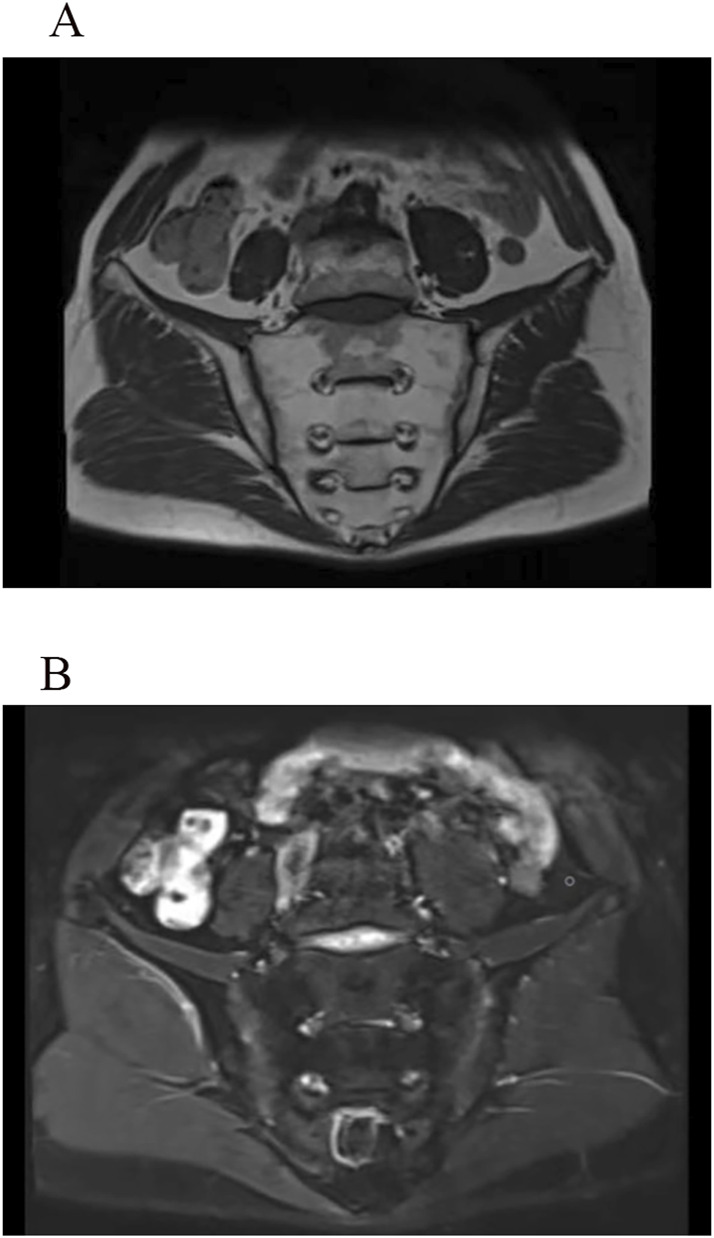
MRI images of the sacroiliac joints show long T1 (A) and T2 (B) signals on both the sacral and iliac surfaces. The articular surfaces appear smooth on T1WI, without signs of bone erosion, new bone formation, or abnormal fat deposition. The joint spaces are normal, with no evidence of pathological narrowing.
